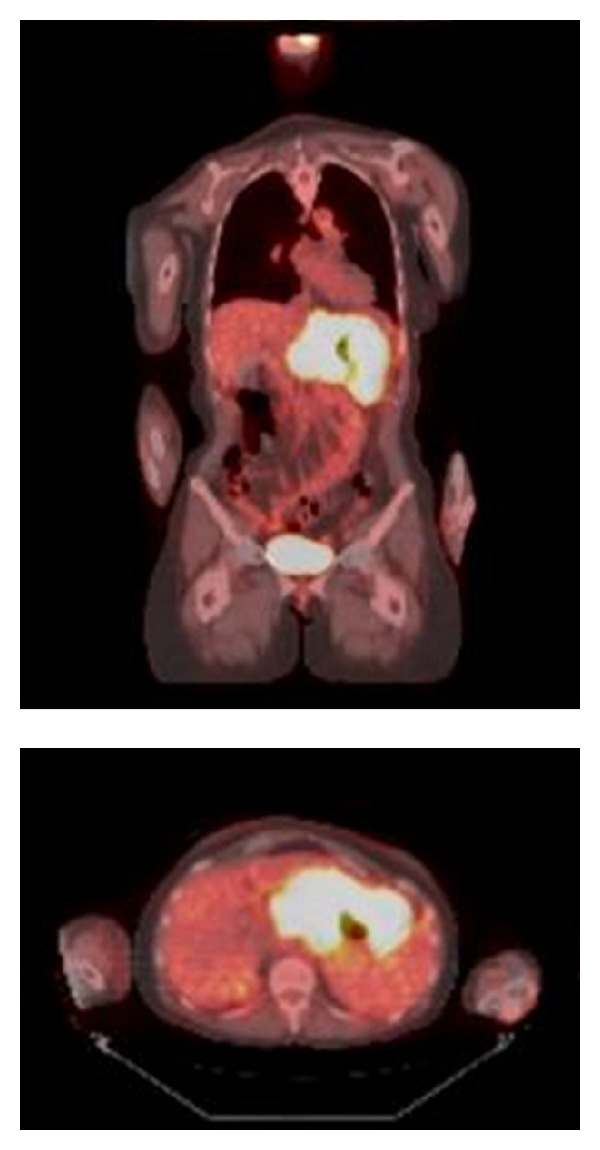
Coronal and cross-sectional PET/CT images demonstrate intense radiotracer uptake in the gastric region.
